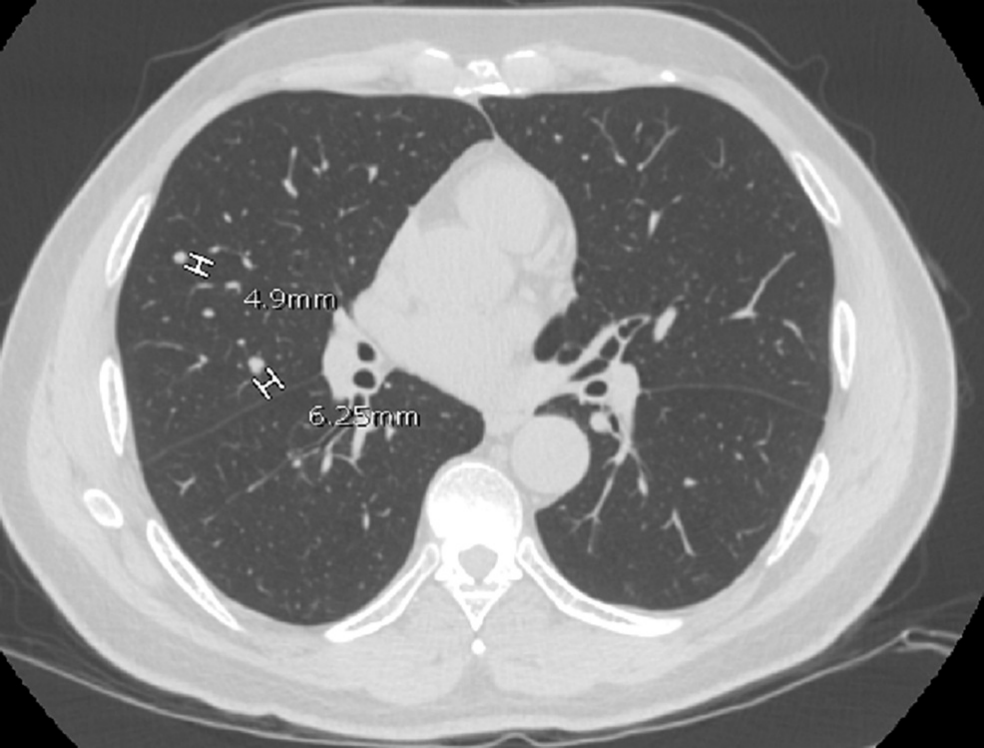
Repeat chest CT scan demonstrating two new RUL nodules
